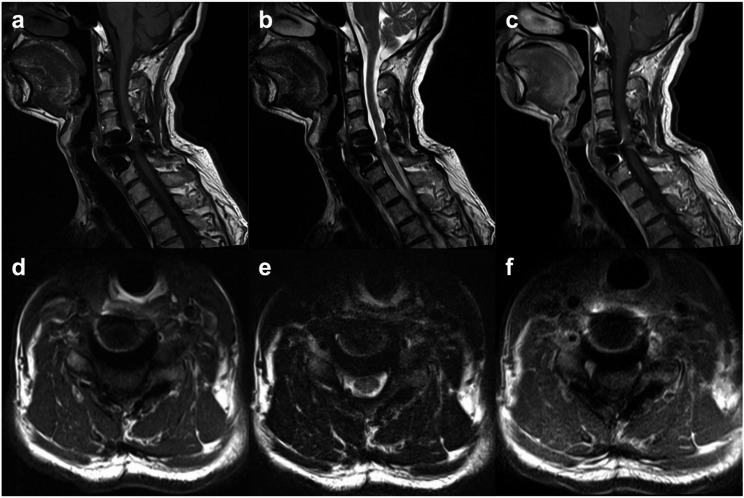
Follow-up MRI at 6 years. a–c Sagittal T1-weighted (a), T2-weighted (b), and post-contrast T1-weighted (c) images show no evidence of residual or recurrent intradural tumour at the C5–6 level. The spinal cord is well decompressed, and the dural contour remains smooth without abnormal enhancement. A susceptibility artifact from the C5–6 artificial disc replacement is visible at the operative level. d–f Corresponding axial T1-weighted (d), T2-weighted (e), and post-contrast T1-weighted (f) images confirm complete tumour removal and resolution of cord compression, with no enhancing lesion in the intradural or foraminal region
